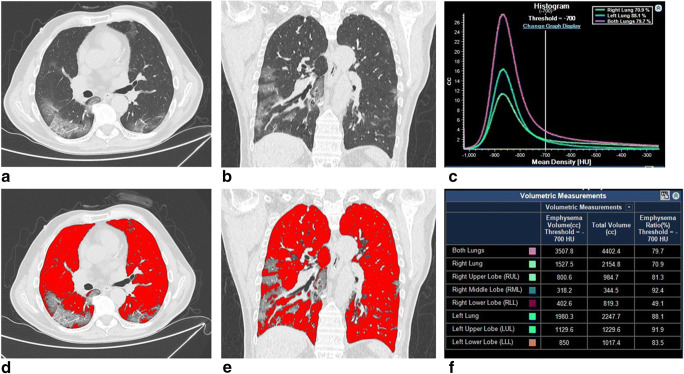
A 61-year-old man with SARS-CoV-2-related pneumonia. CT images in axial (a) and coronal (b) planes, with window width and level for the evaluation of the lung parenchyma. The images show the presence of bilateral ground-glass opacities and partial crazy-paving pattern. Final CT score: 12. Post-processed CT images in axial (d) and coronal (e) sections showing low attenuation areas based on thresholds of − 700 HU that correspond to ventilated lung volume (VLV). CT density histogram based on the attenuation lung analysis showing both long volumes that correspond to TLV (purple line), left (blue line), and right (green line) lung volumes (c). Post-processing volumes of different lung segments calculated by the software (f)
